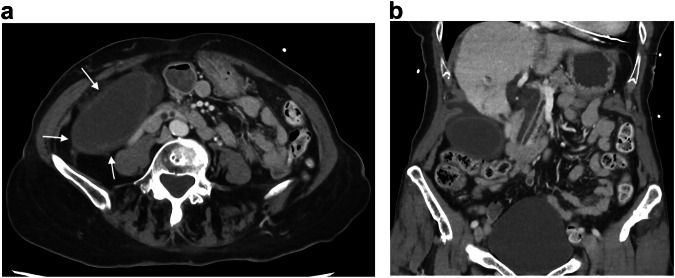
Volvulus of the gallbladder. Axial and coronal portal phase CT (a, b) in an 84-year-old woman shows dilatation of the gallbladder and horizontal rotation of its axis with poor enhancement of its walls (arrows)
